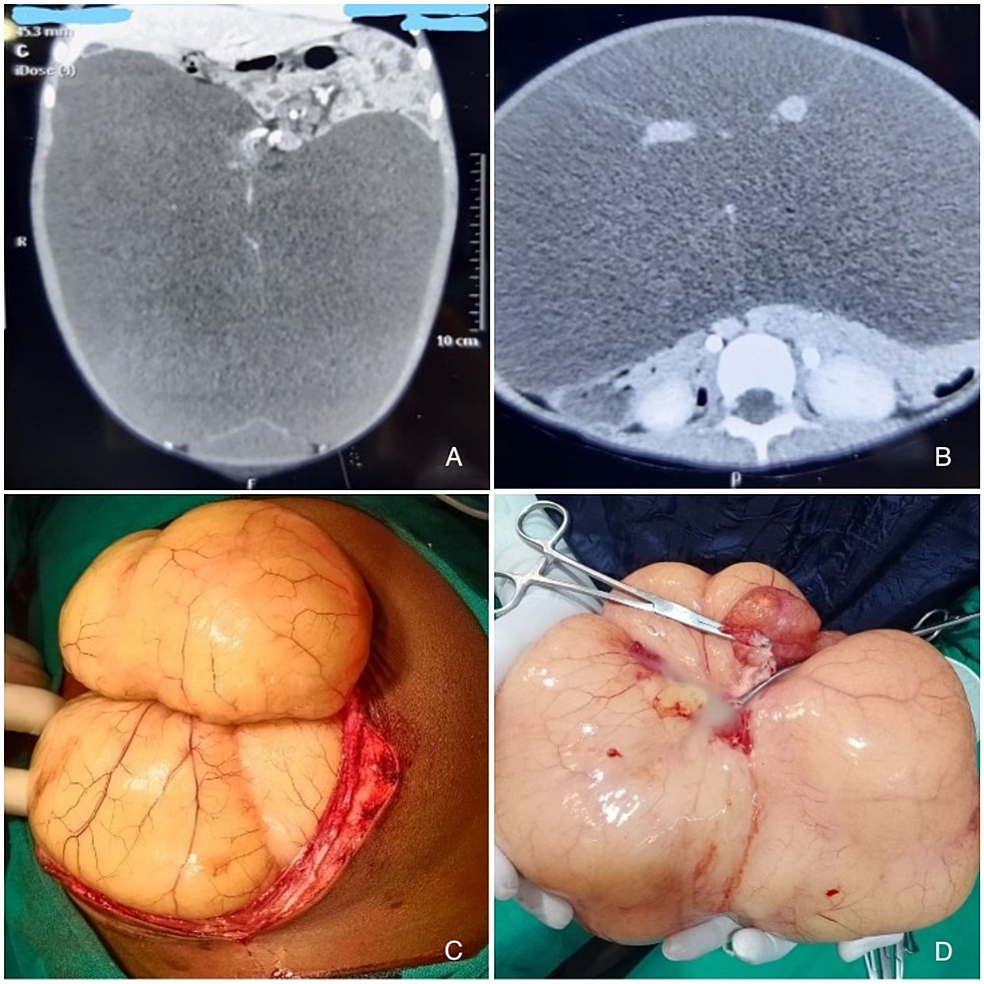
CECT and intra-operative images of mesenteric cyst. (A and B) Contrast-enhanced computed tomography images of the abdomen showed a large fluid-filled cyst occupying the entire abdominal cavity. The bowel loops were pushed aside. (C) Intraoperative bulging out of the cyst from the abdominal incision. (D) Excised cyst with a loop of the attached duodenal segment. CECT: Contrast-enhanced computed tomography
